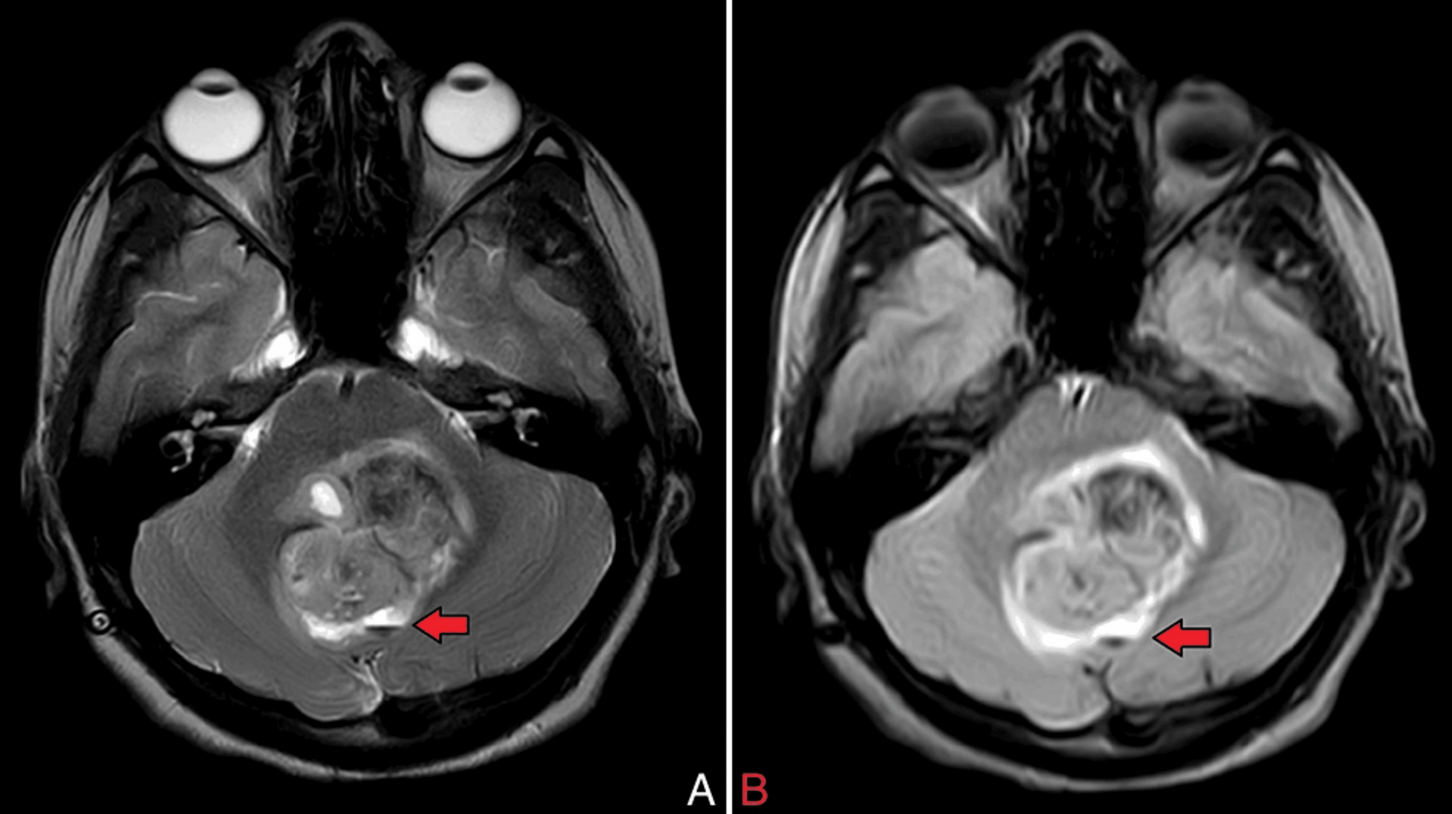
A) Axial T2 and B) FLAIR MRI brain images The red arrow shows that the lesion appears hyperintense with perilesional oedema. FLAIR: Fluid-attenuated inversion recovery; MRI: Magnetic resonance imaging
